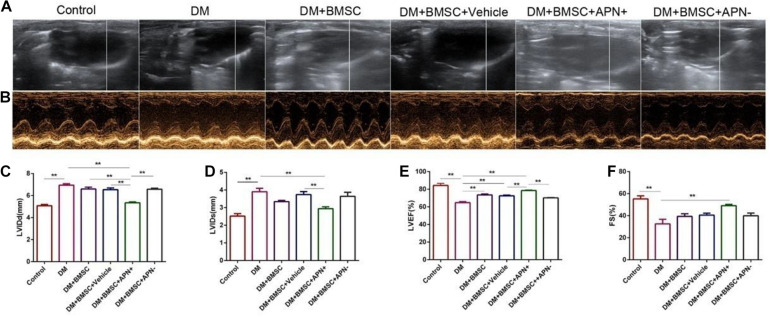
Effects of APN on diabetes-induced LV dysfunction. (A) Two-dimensional echocardiograms. (B) M-mode echocardiograms. (C) Left ventricular internal diameter at end-diastole (LVIDD). (D) Left ventricular internal dimension systole (LVIDs). (E) LV ejection fraction (LVEF). (F) Fractional shortening (FS). Data are mean ± SD; p* < 0.05, *p* < 0.01.
